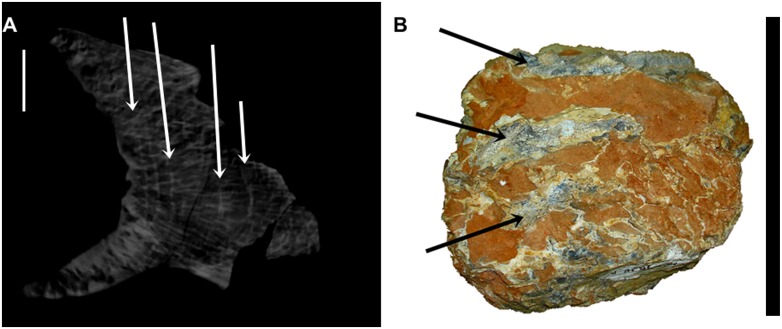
The camellate rings revealed by tomographic CT scans in cervical vertebrae. (A) The camellate rings Cv13 evidenced by the white arrows. Those structures are interpreted as growth structures. (B) The intercalation of external high density tissue (black arrows) and internal pneumatic tissue in an isolated fragment of a cervical vertebra. Scale bar: 50mm.
